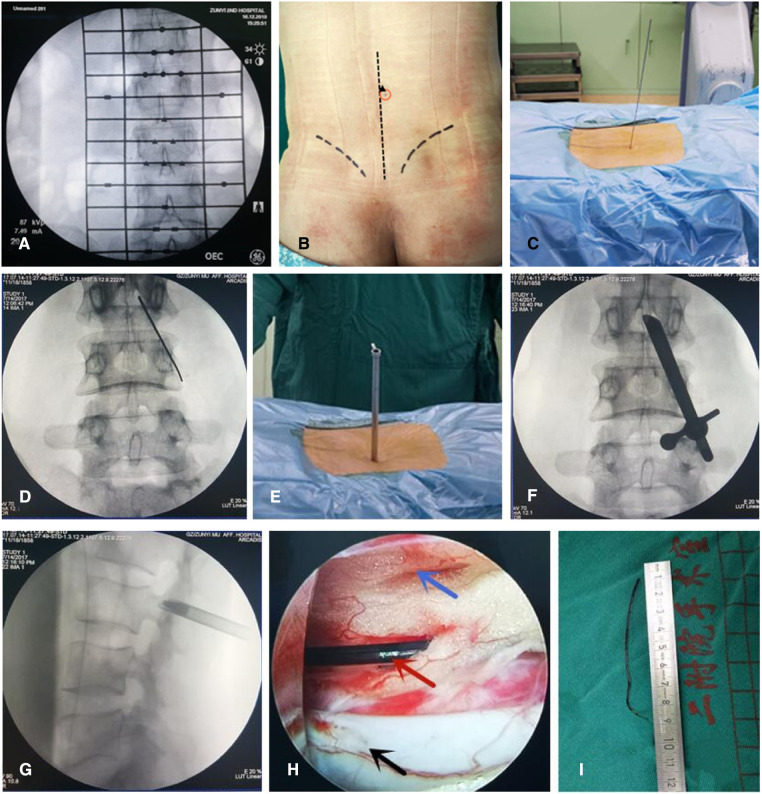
Typical case: Female, 26 years old, cesarean section, lumbar 2-3 epidural space cephalic catheter rupture and residual epidural catheter. (A,B) x-ray was used to locate the puncture gap and mark the puncture point; (C) a puncture guide needle was inserted at the marked point; (D) x-ray fluoroscopy was used to determine the correct position of the guide needle; (E) a working sleeve was placed; (F,G) x-ray fluoroscopy was performed to ensure that the working sleeve was positioned correctly and that the depth was appropriate; (H) The residual epidural catheter was clearly visible under the endoscopic vie; (I) Remove the remaining epidural catheter completely.
